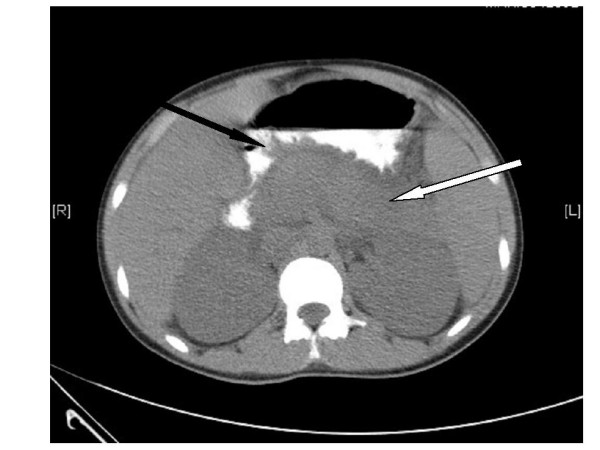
Non contrast computed tomography (CT) scan of the abdomen showing a bulky pancreas (white arrow) with peri-pancreatic fat stranding (black arrow) suggestive of acute pancreatitis.
